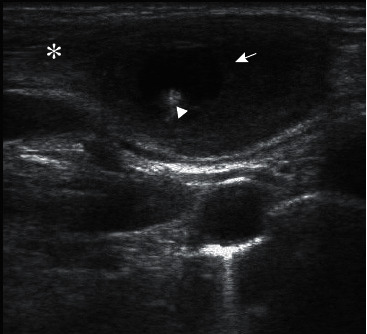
A 20-year-female who presented with gradual neck swelling and mild pain for 4 months. Sagittal grayscale ultrasonography image showing well-defined thick-walled cystic lesion (arrow) in the intramuscular plane in the distal SCM (asterisk) with eccentric hyperechoic foci (arrowhead) within the cavity and surrounding edematous changes.
